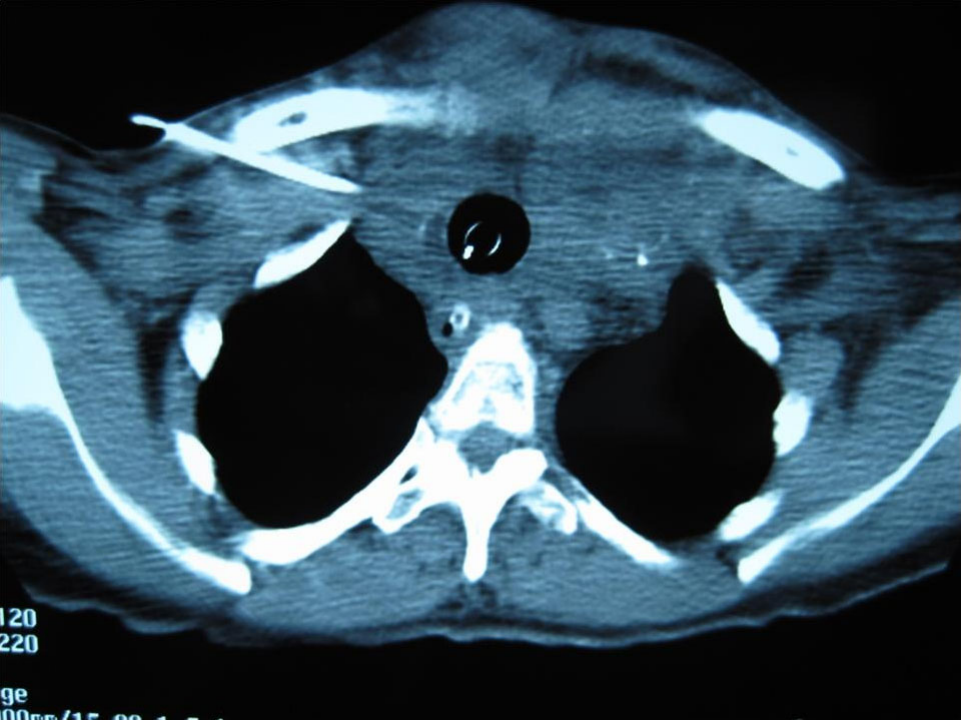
A computed tomography (CT) scan at the level of T2 showing the endotracheal tube situated within the tracheal lumen. The tracheal diameter was 2 cm at this level. No retrosternal tracheal compression was evident as compared to Figure 2. There was mild retrosternal extension on the left down to the level of the great vessels. A central venous line was noted on the right.
